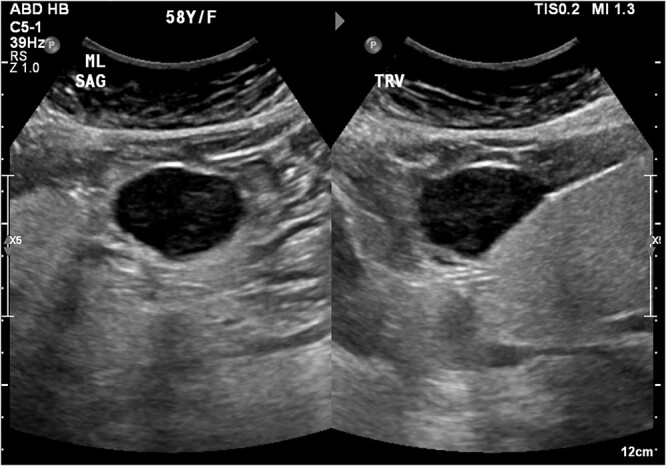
Homogonous hypoechoic lesion anterior to the left hepatic lobe. Color doppler images (not shown) shows internal blood flow.
